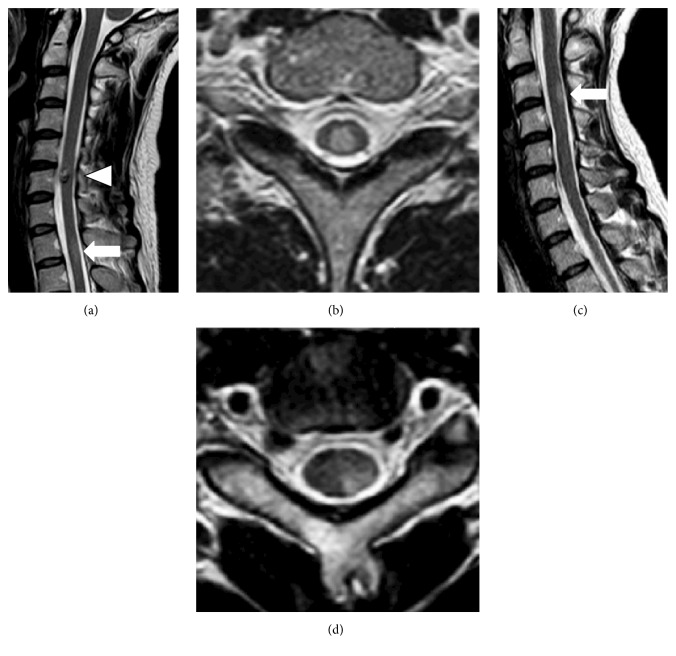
MRI images of atopic myelitis. (a) Cavernous angioma, which exhibits low intensity signal around the ventral side of the spinal cord at C6 vertebral level, is seen on T2WI (arrowhead). An intramedullary high-signal intensity area is found on the thoracic spinal cord at T1 vertebral level (arrow). (b) Axial image at T1 vertebral level on T2WI. An intramedullary change in signal intensity is observed on both sides of the spinal cord and around the dorsal side of the spinal cord. (c) A new intramedullary high-signal intensity area on T2WI is found on the dorsal side of the cervical spinal cord at C3 vertebral level (arrow). (d) Axial image at C3 vertebral level on T2WI. The lesion is locally observed on the left posterior funiculus.
